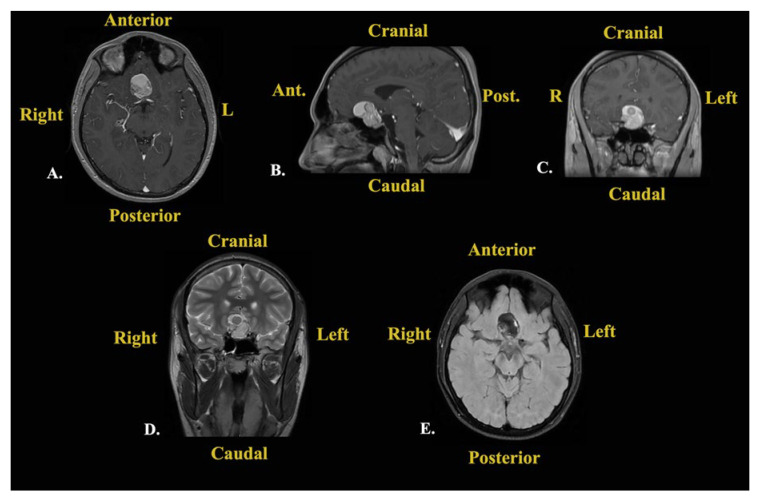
Preoperative contrast-enhanced brain MRI: (A) axial T1 contrast-enhanced Fast Spoiled Gradient-Echo (FSPGR) sequence; (B) sagittal contrast-enhanced T1-weighted multiplanar reformation/reconstruction (MPR) sequence; (C) coronal contrast-enhanced T1-weighted MPR sequence; (D) coronal T2-weighted FSE sequence; (E) axial T2-weighted FLAIR FS sequence.
